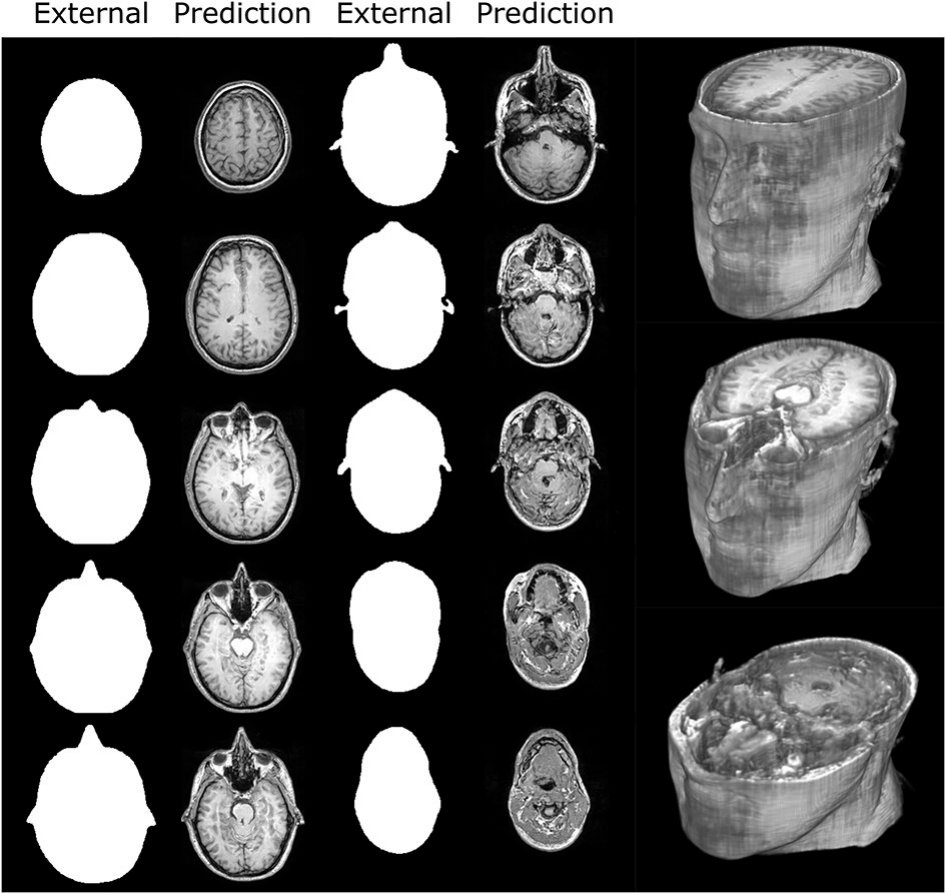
Examples of the external mask slices and corresponding predicted MRI data obtained using photogrammetry of a human subject. Some volumetric renders are shown on the right with cutaways at three different axial levels
